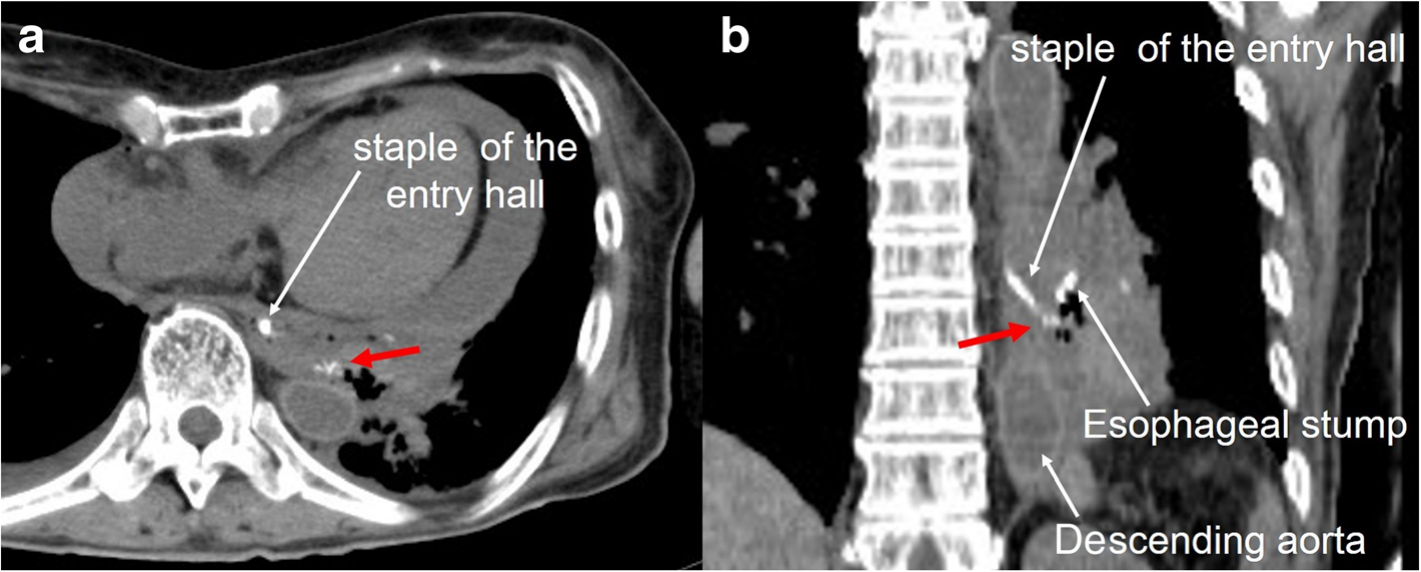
Post-mortem computed tomography showing that the dorsal stapled edge (red arrow) of the entry hole was in direct contact with the bare descending aorta. a Axial plane. b Coronal plane
